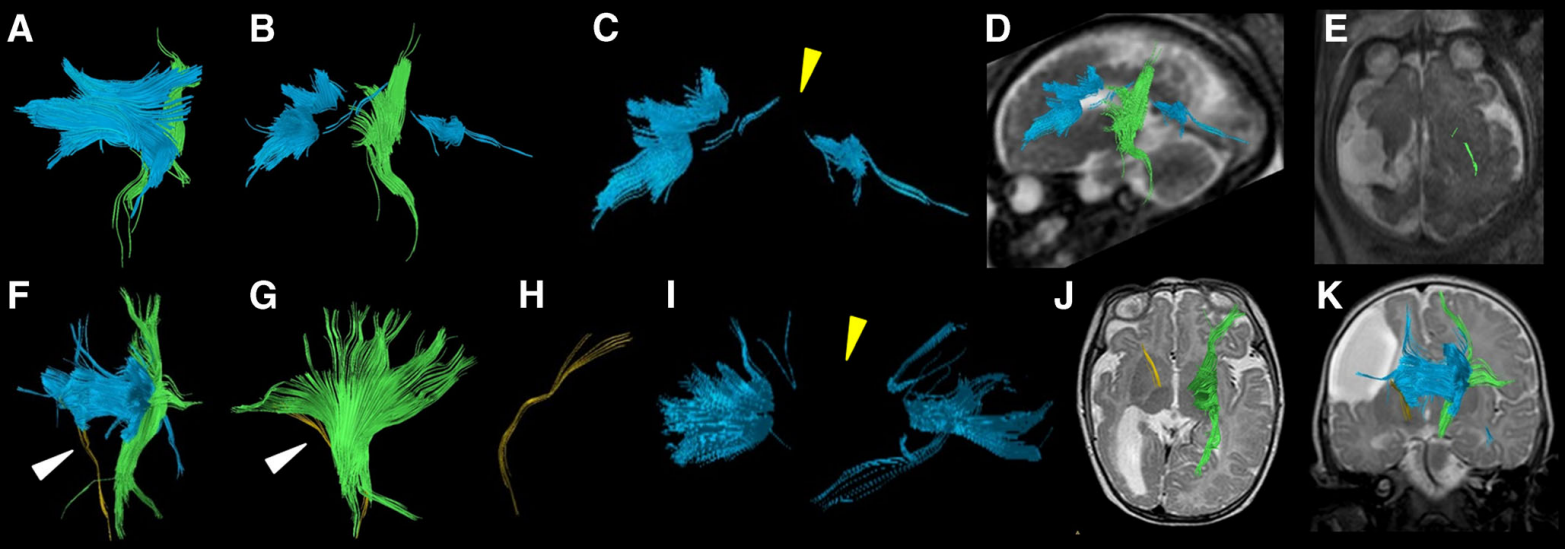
Fetal and postnatal diffusion tensor imaging corticospinal tract segmentation in a boy with right middle cerebral artery territory infarct (case 12). a–e Age 33+6 gestational weeks. a Coronal view of the segmentation shows the corpus callosum (*blue*) and left corticospinal tracts but not the right corticospinal tract. b Sagittal view shows the corpus callosum (*blue*) and left corticospinal tract (*green*). c Sagittal view of the corpus callosum reveals a paucity of tracts in the body segment of the corpus callosum (*yellow arrowhead*). d Overlay of the left corticospinal tract (*green*) and corpus callosum (*blue*) on a sagittal T2-weighted image is shown for orientation. e The left corticospinal tract traverses the expected region of the posterior third of the posterior limb of the internal capsule on axial T2-weighted MR image. f–k Age 4 months. f Coronal view of the segmentation shows the corpus callosum (*blue*), intact left corticospinal tract (*green*) and very few sparse fibers in the region of the right corticospinal tract (*arrowhead*), presumably virtually absent from significant right middle cerebral artery territory infarct. g Sagittal view of the intact left (*green*) and residual right (*arrowhead*) corticospinal tracts. h Sagittal view of the right corticospinal tract only (*yellow*). i Sagittal view of the corpus callosum reveals a paucity of tracts involving the body of the corpus callosum (*yellow arrowhead*), which is similar to the prenatal tractography (c). j Overlay of the segmented bilateral corticospinal tracts (*yellow*, right; *green*, left) on axial T2-weighted MR image for orientation shows the tracts traversing the expected region of the posterior third of the posterior limb of the internal capsule. k Overlay of the segmented bilateral corticospinal tracts (*yellow*, right; *green*, left) on coronal T2-weighted image for orientation
